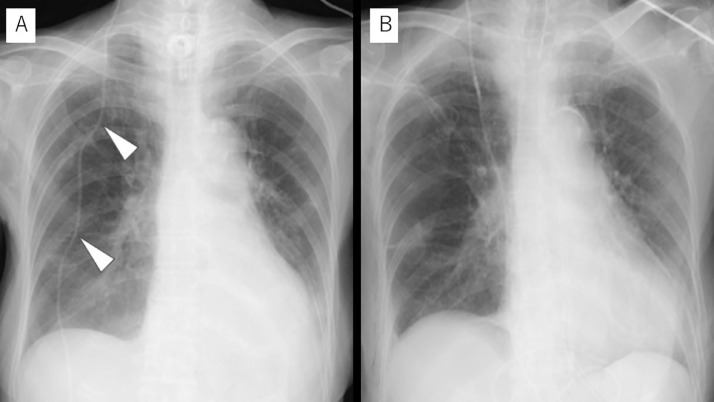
Chest radiographs after the first ventriculoperitoneal shunt procedure (A) and after the shunt revision (B). Note that the tube bends twice at the chest wall (white arrowheads), which we call the “double bending sign” on (A), whereas the tube has no bend in (B).
